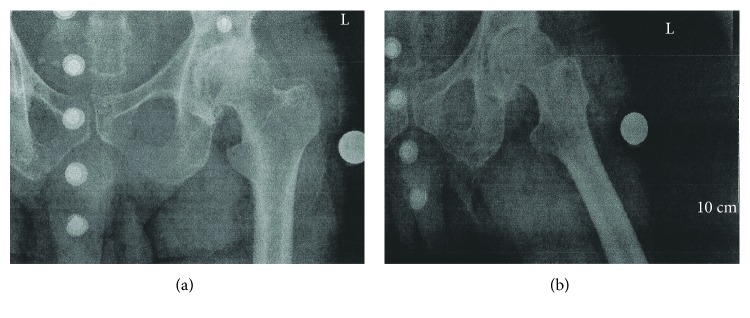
Preoperative AP and lateral X-rays showing stage IV osteoarthritis of the left hip.
